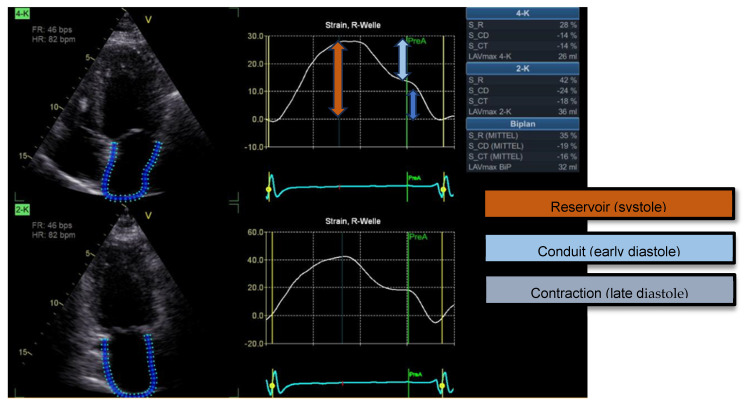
Example of left atrial strain analysis using speckle tracking echocardiography.
